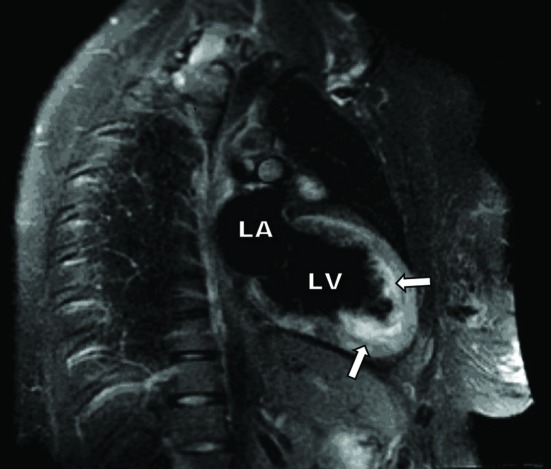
Sagital view of the heart on Cardiac MRI with T2 weighted and viability/scar imaging showing diffuse circumferential, abnormal myocardial enhancement consistent with myocarditis of the left ventricle (shown by arrows).
